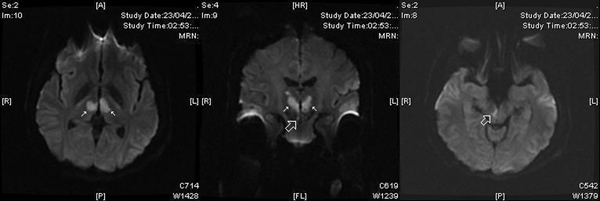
Axial (right and left) and coronal (center) trace diffusion-weighted magnetic resonance images, obtained 20 hours after the onset of symptoms, show bilateral thalamic areas of high signal intensity (white arrows) compatible with acute bilateral paramedian thalamic infarcts. Rostral midbrain infarct is limited to right periaqueductal gray matter (empty white arrows).
